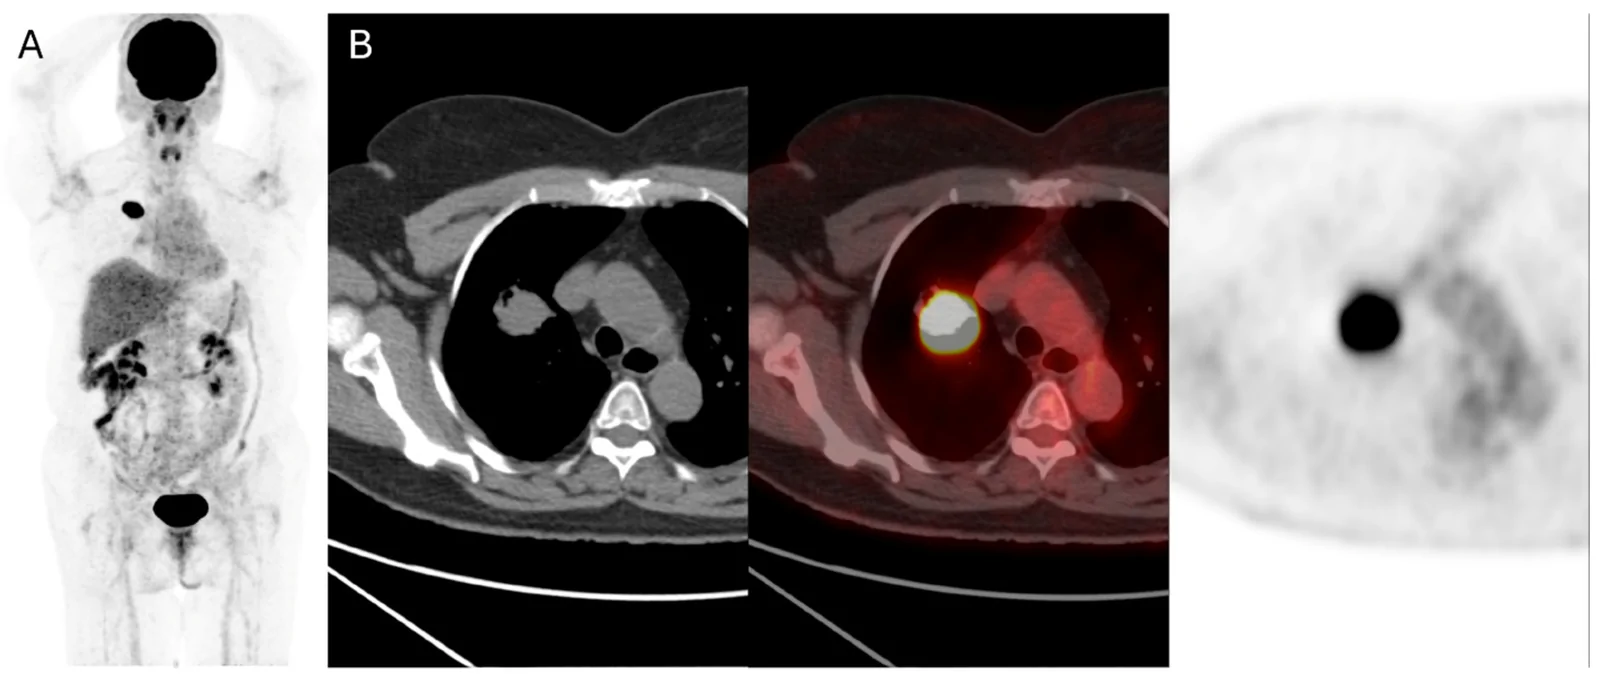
Baseline 18F-FDG PET/CT demonstrating intense metabolic activity in the right upper lobe lesion. (A) Whole-body maximum intensity projection (MIP) demonstrating an isolated hypermetabolic lesion in the right upper lung field, with no evidence of extrathoracic 18F-FDG-avid disease. (B) Axial CT, fused PET/CT, and PET images confirming a well-defined right upper lobe pulmonary nodule exhibiting intense 18F-FDG uptake, consistent with a metabolically active primary lung tumor.
